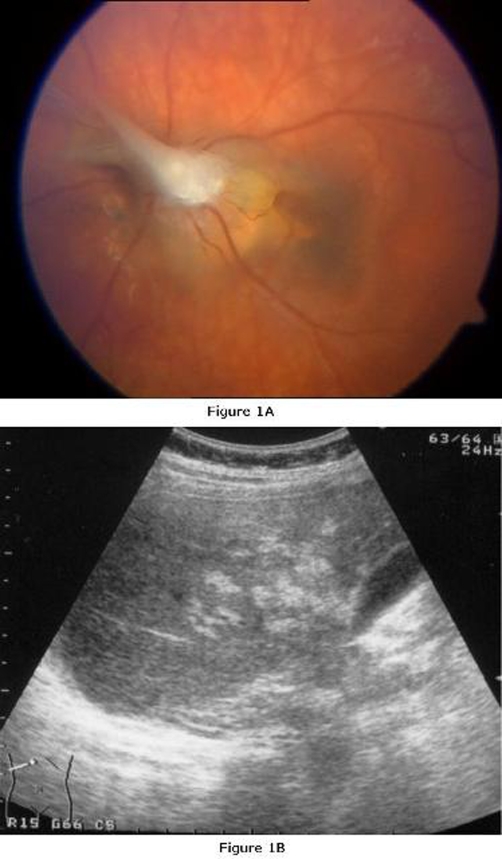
(A) Sickle-shaped epiretinal membrane and inactive post-inflammatory retinal scar of the left eye in the 8-year-old child infected with *T. canis,* as visualized using direct ophthalmoscopy. (B) Multiple hepatic granulomas in the 8-year-old child infected with *T. canis*. An ultrasound of the abdomen is shown.
